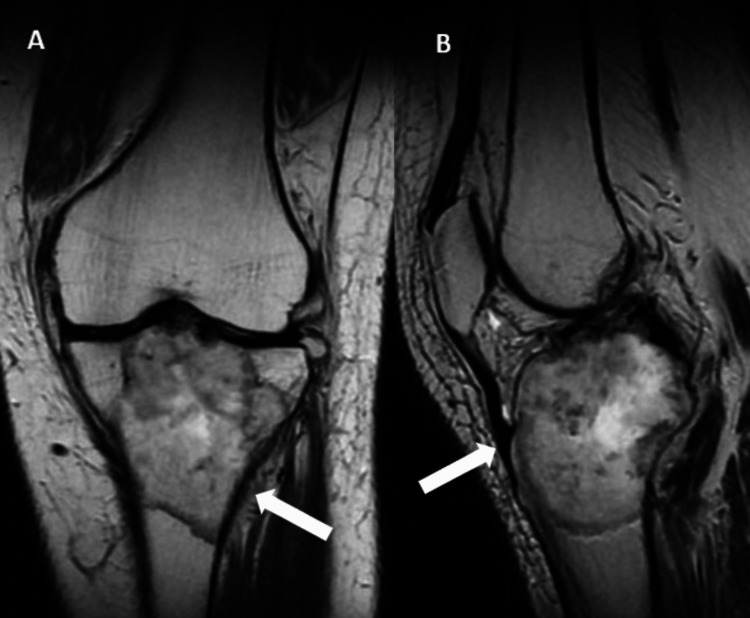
MRI scan of the left knee A and B show cortical breach and soft tissue shadow in the proximal tibia MRI: magnetic resonance imaging
